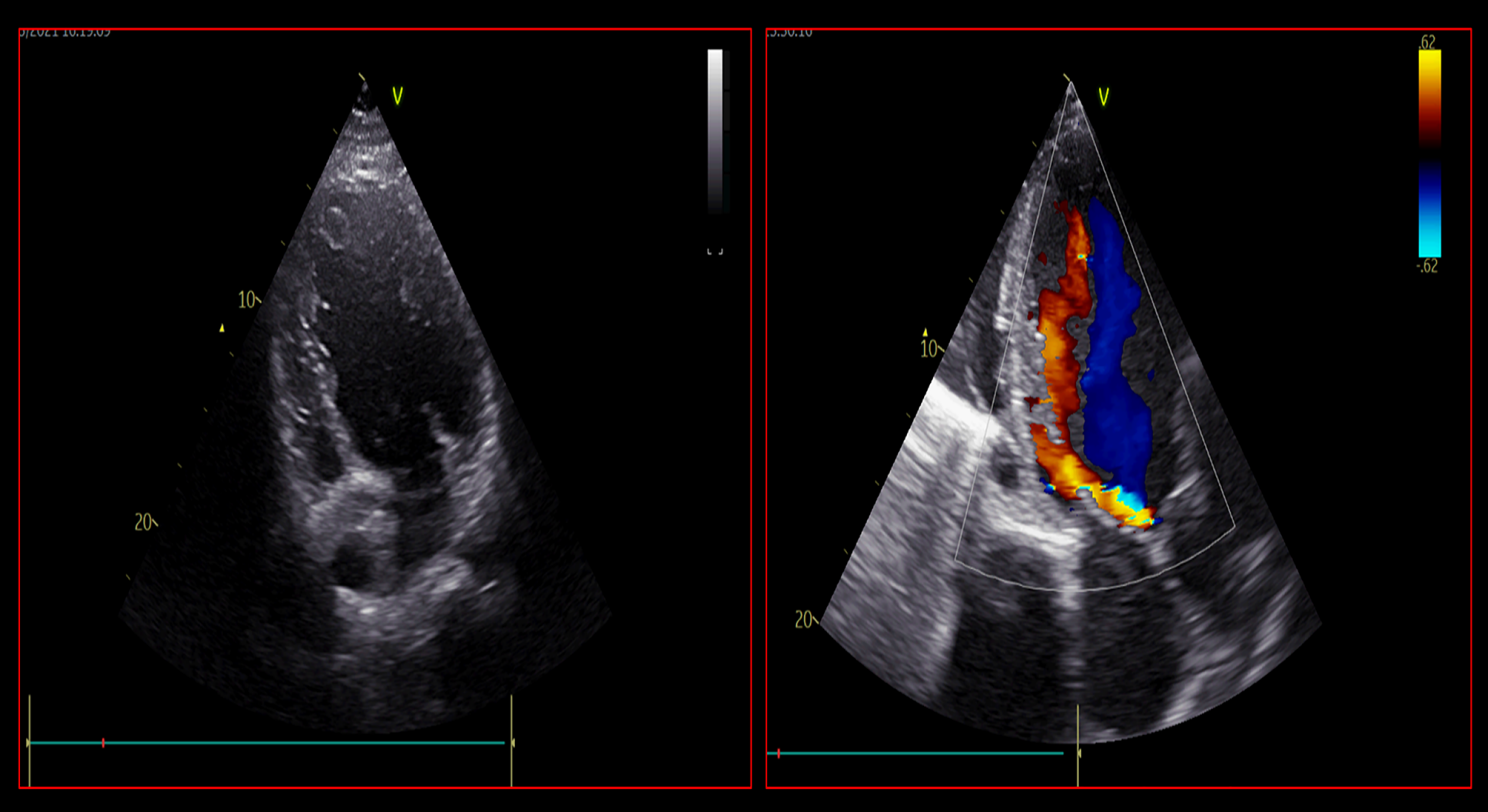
Echocardiographic assessment of possible contraindications to mechanical circulatory support. The picture on the left shows the presence of intracardiac thrombosis, localized at the apex of left ventricle. On the other hand, the picture on the right shows a case of significant aortic regurgitation with an eccentric jet, visualized in apical 3-chamber view. Both of these patients presented two possible contraindications to mechanical circulatory support placement
